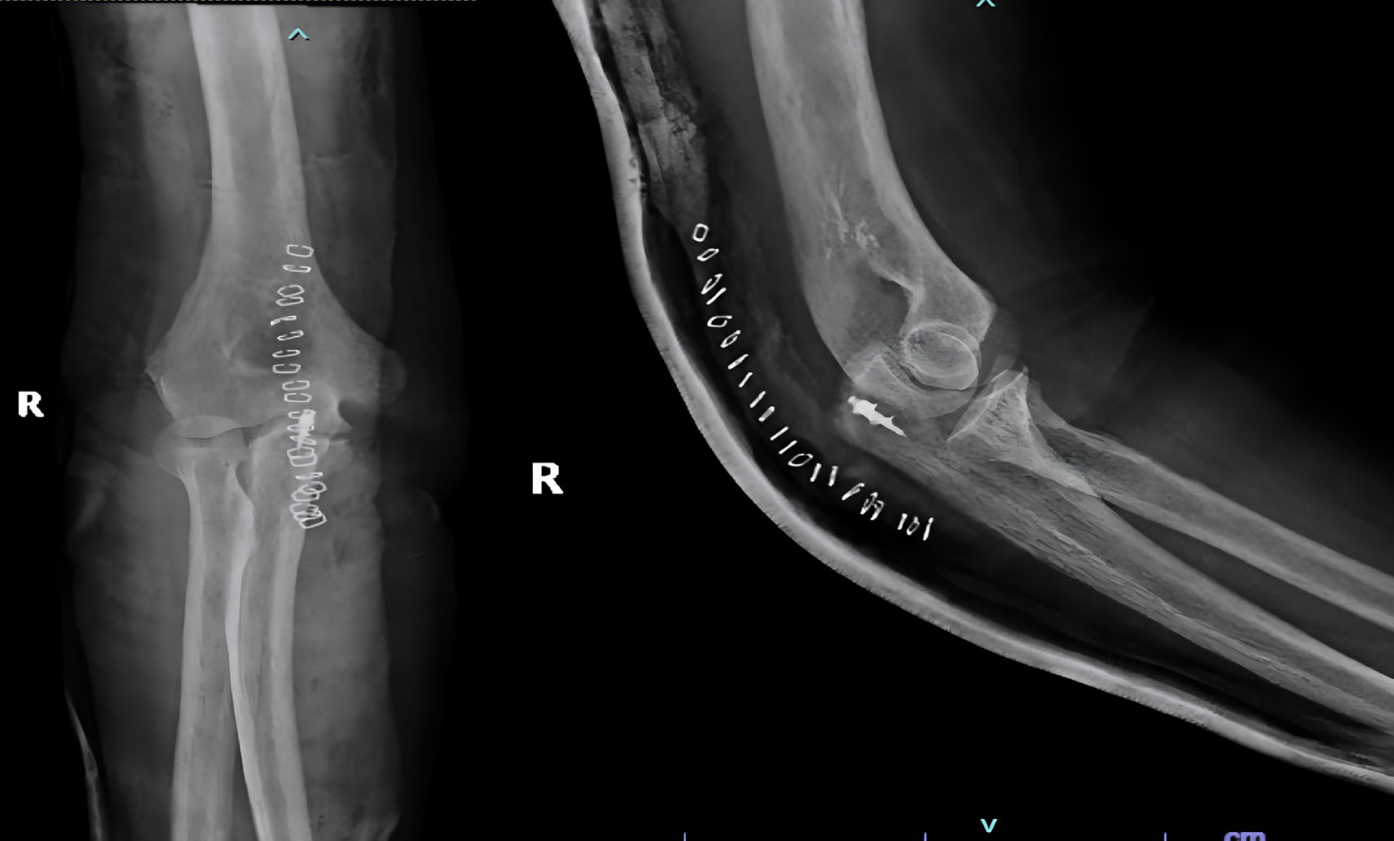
Post-operative radiographs after fixation of tensons using suture anchors.
